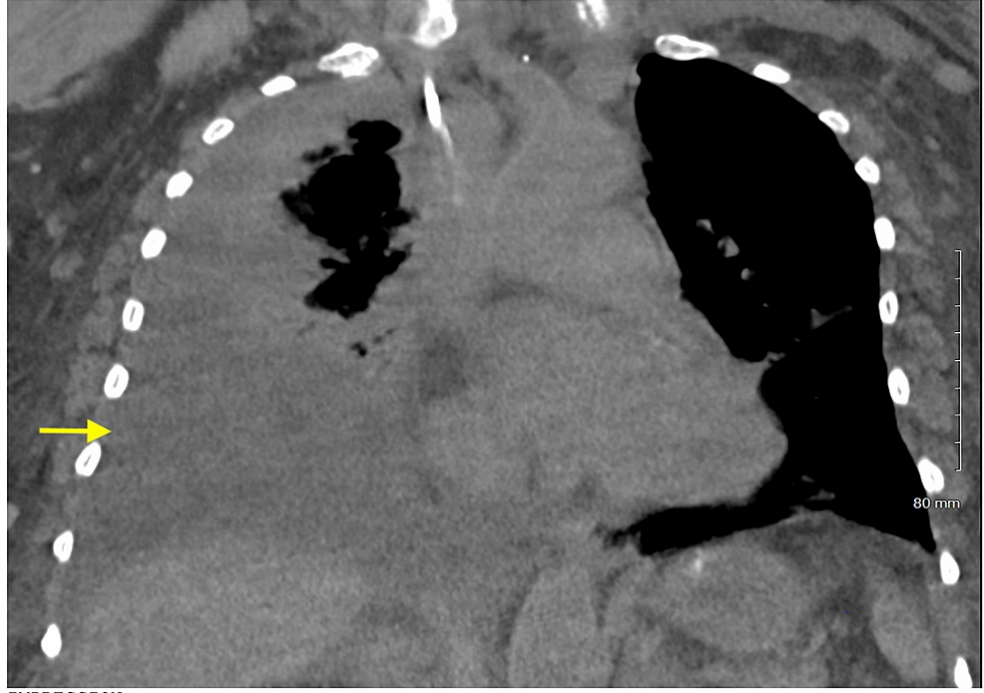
CT scan showing large right pleural effusion
